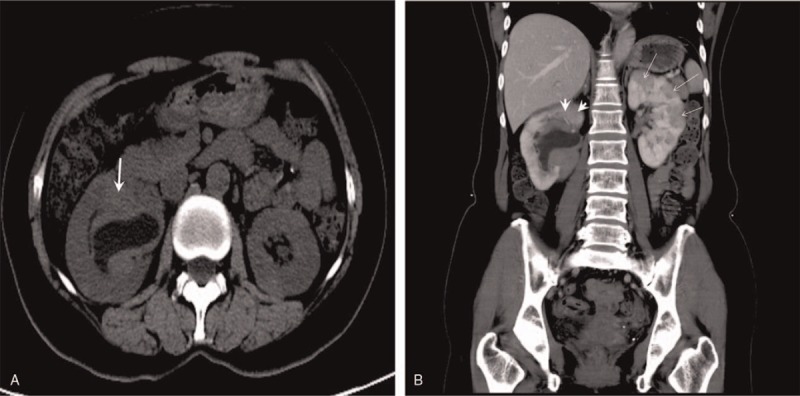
IgG4-RKD presentation on CT, displayed as a transaxial image precontrast (A) and coronal multiplanar reformation postcontrast (B). Precontrast image shows that the renal parenchyma lesions were not clear and that the right pelvic mass was slightly hyperdensity (thick arrow). Postcontrast image showed the left multiple renal parenchyma lesions were round, wedge-shaped, and with mild enhancement (arrows); the right renal parenchyma lesion showed diffuse patchy infiltration (arrowheads). CT = computed tomography, IgG4-RKD = IgG4-related kidney disease.
